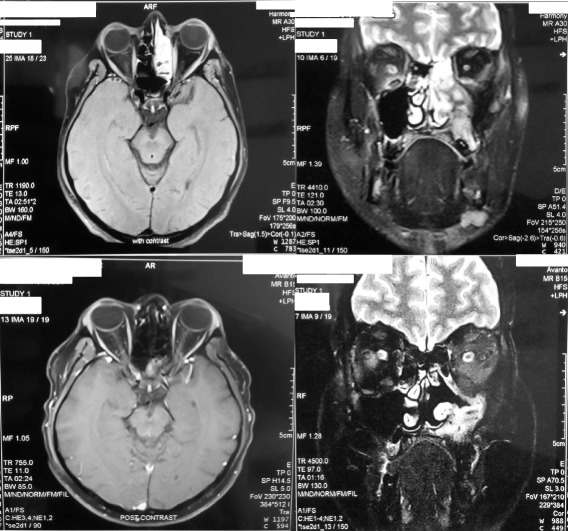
Magnetic resonance images show response with combination therapy of methotrexate and prednisolone. Top row - Imaging reveals the recurrence of tumour in the left temporal region and infra temporal fossa with involvement of the sinuses [left maxillary antrum projecting into the middle meatus of the nose, sphenoidal and ethmoid sinus] and involvement of the recti muscles of the left orbit. Bottom row- Imaging demonstrates absence of tumour in the temporal region and orbit and clearance within the middle meatus. Though reduced, persistence of residual tumour within the left maxillary antrum is seen.
